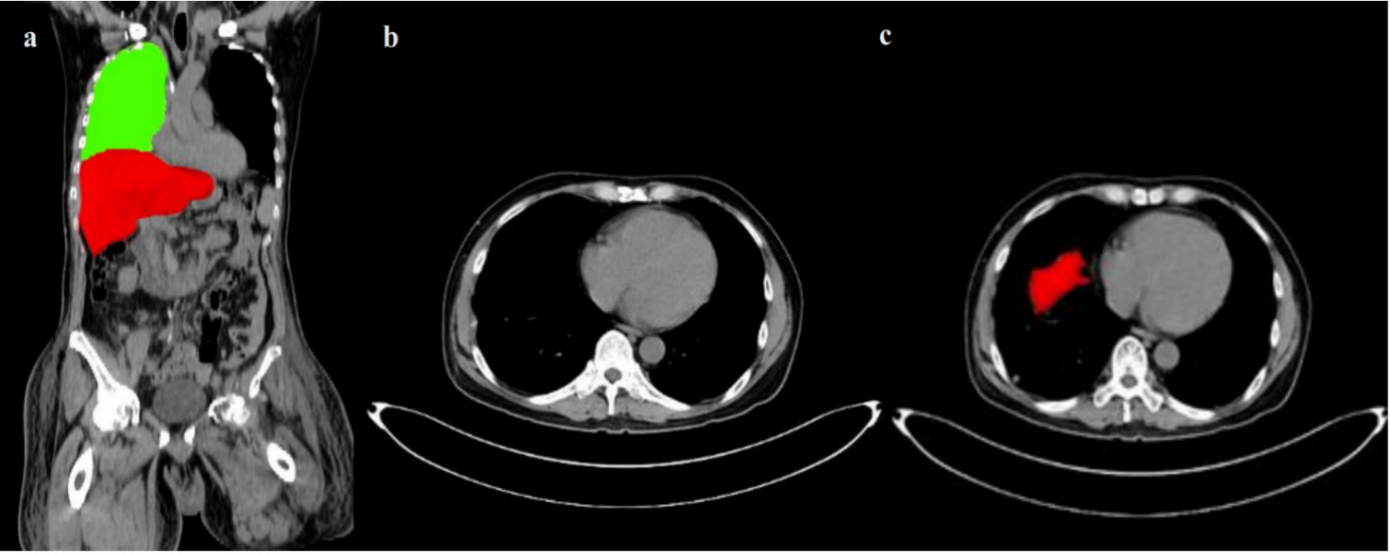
Liver location with respect to lung (green) (a), CT slice just before liver dome (b) detected liver dome in red (c)
